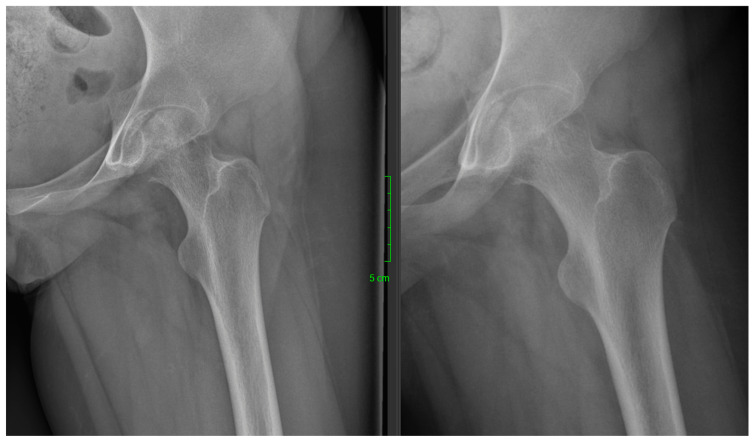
Postoperative AP hip joint radiographs taken at 6 and 12 months.
